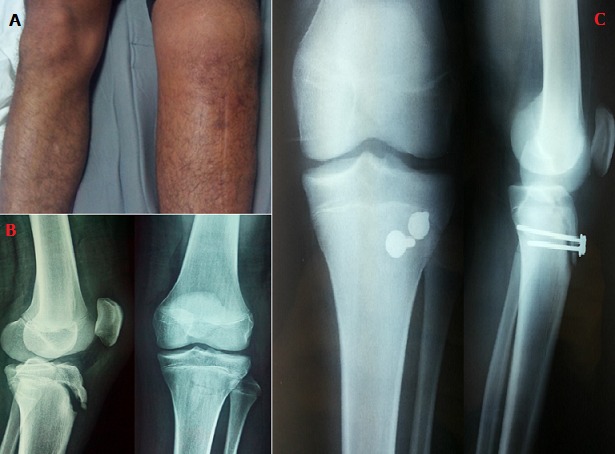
(A) clinical aspect of an anterior tibial tuberosity removal: large left knee oedematous + deficit of active extension of the same knee; (B) standard radiography of the left knee: avulsion of the anterior tibial tuberosity; (C) radiography of postoperative control: reduction + osteosynthesis by two cortical screws
